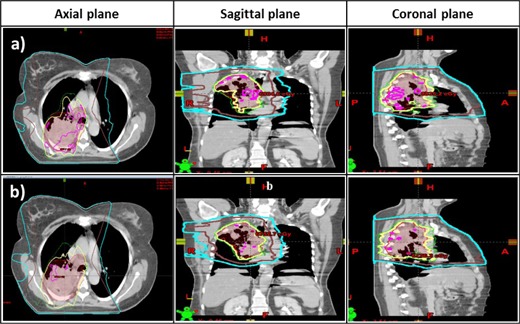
Final dose distribution for a free‐breathing IMRT plan (Patient #1) for a lung tumor superimposed on CT images in the axial, sagittal, and coronal plane for (a) the original plan without the intermediate dose calculation module, and for (b) the new plan with the intermediate dose calculation module. Isodose lines for 105% (purple), 100% (yellow), and 95% (green) of the prescription dose and 10 Gy (brown), and 5 Gy (cyan) are shown. Note the increased homogeneity in the target, which corresponds to a decrease in hot spots in the new plan.
